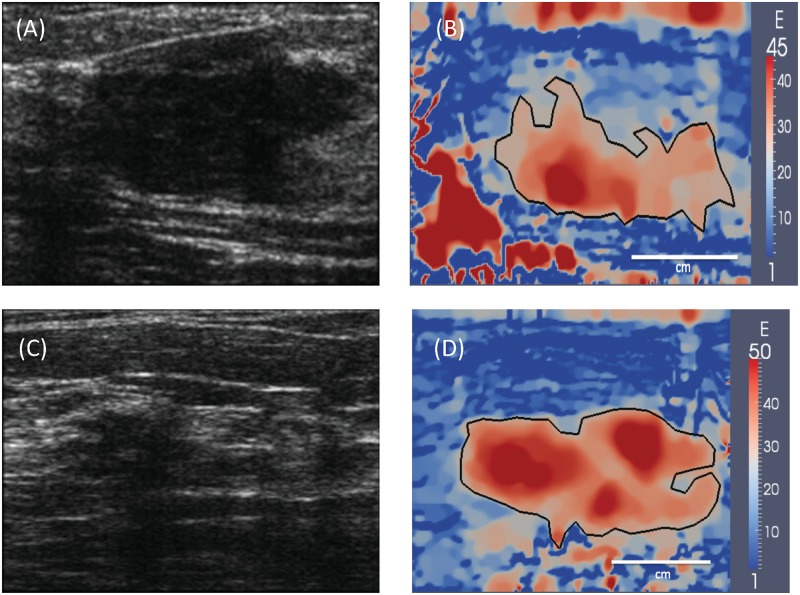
(A) and (C): B-mode ultrasound images of two typical invasive ductal carcinomas. (B) and (D): Corresponding Young’s modulus images generated using elasticity imaging. The tumor boundary is represented by a black curve that is drawn using 50 peak tumor modulus value. The modulus distribution within the tumors is heterogeneous and the margins of the tumors are rough.
